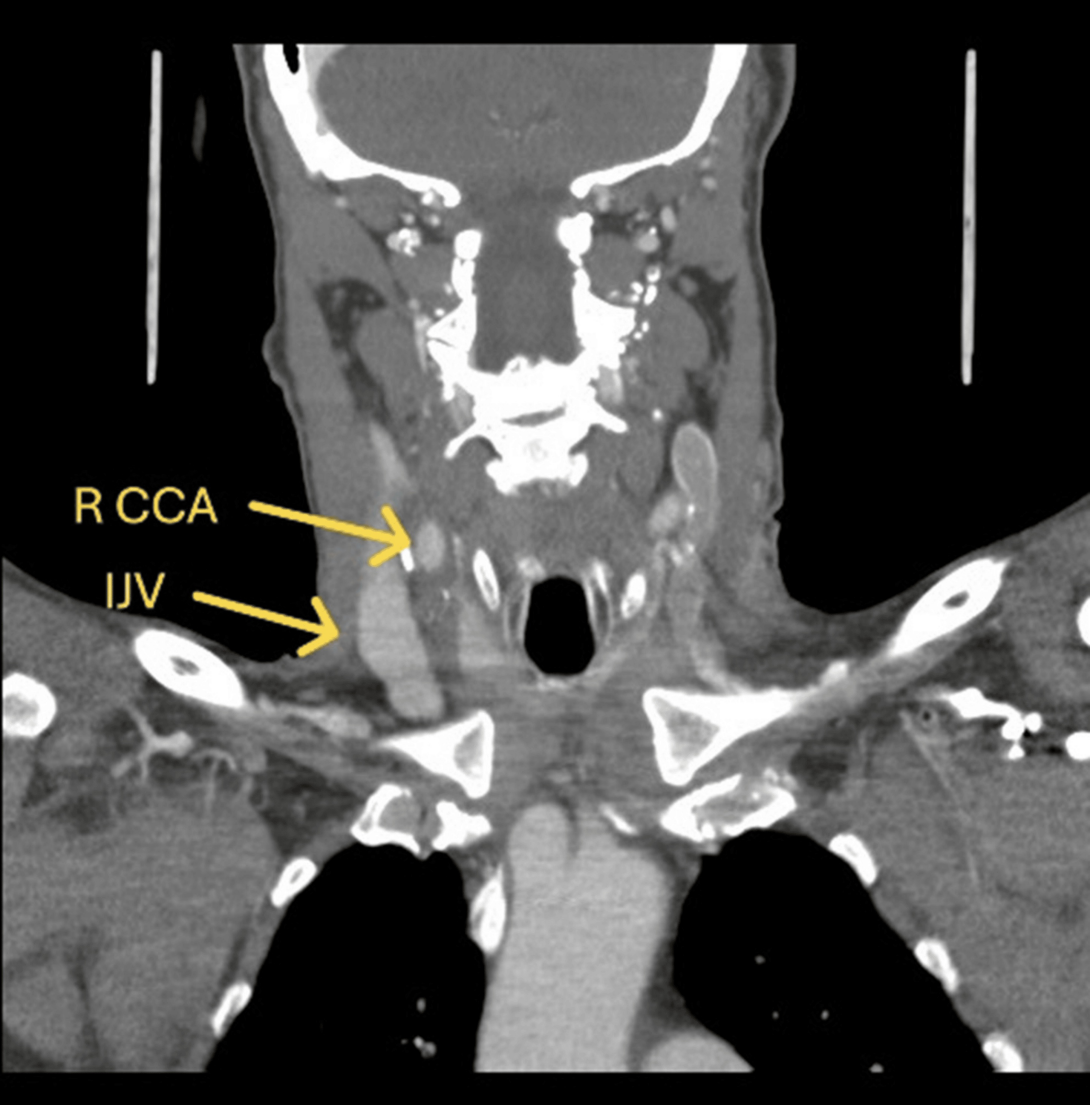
Postoperative computed tomography-angiography showing no persistent fistula R CCA: right common carotid artery; IJV: internal jugular vein
